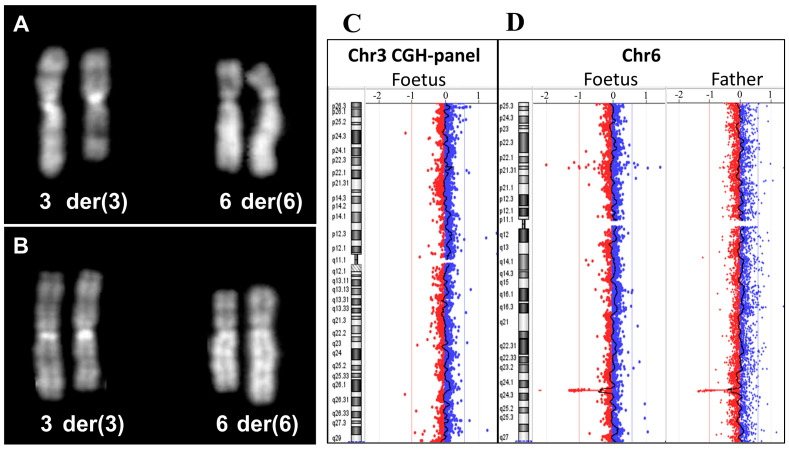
A 42-year-old G3P0 pregnant woman (2 first trimester miscarriages) was seeking prenatal diagnosis due to advanced age. On the first ultrasound, there was an increased nuchal translucency, 3.2 mm, while the general development was in the normal range. The combined test (PAPP-A and free beta-HCG) resulted in a high risk for trisomy 21, 1:5, and trisomy 13, 1:62. A chorionic villus sampling was performed at 12.5 weeks gestational age from cytotrophoblasts and after cultures of mesenchymal tissue; a male karyotype was observed with an apparently balanced translocation involving chromosomes 3 and 6 (A). The extension of the chromosomal analysis to parents showed the same translocation in the paternal karyotype and allowed us to define the breakpoints in 3q25.1 and 6q24.2 (B). The maternal karyotype was normal. At the same time, a CGH array was performed during a placental biopsy and deletion was evident: arr[GRCh37] 6q24.2(143512294_144136217,)x1 (C,D). The deletion was heterozygous, at a size of 624 Kb of chromosome 6 at cytoband q24.2, and it included the OMIM gene *PEX3*.
